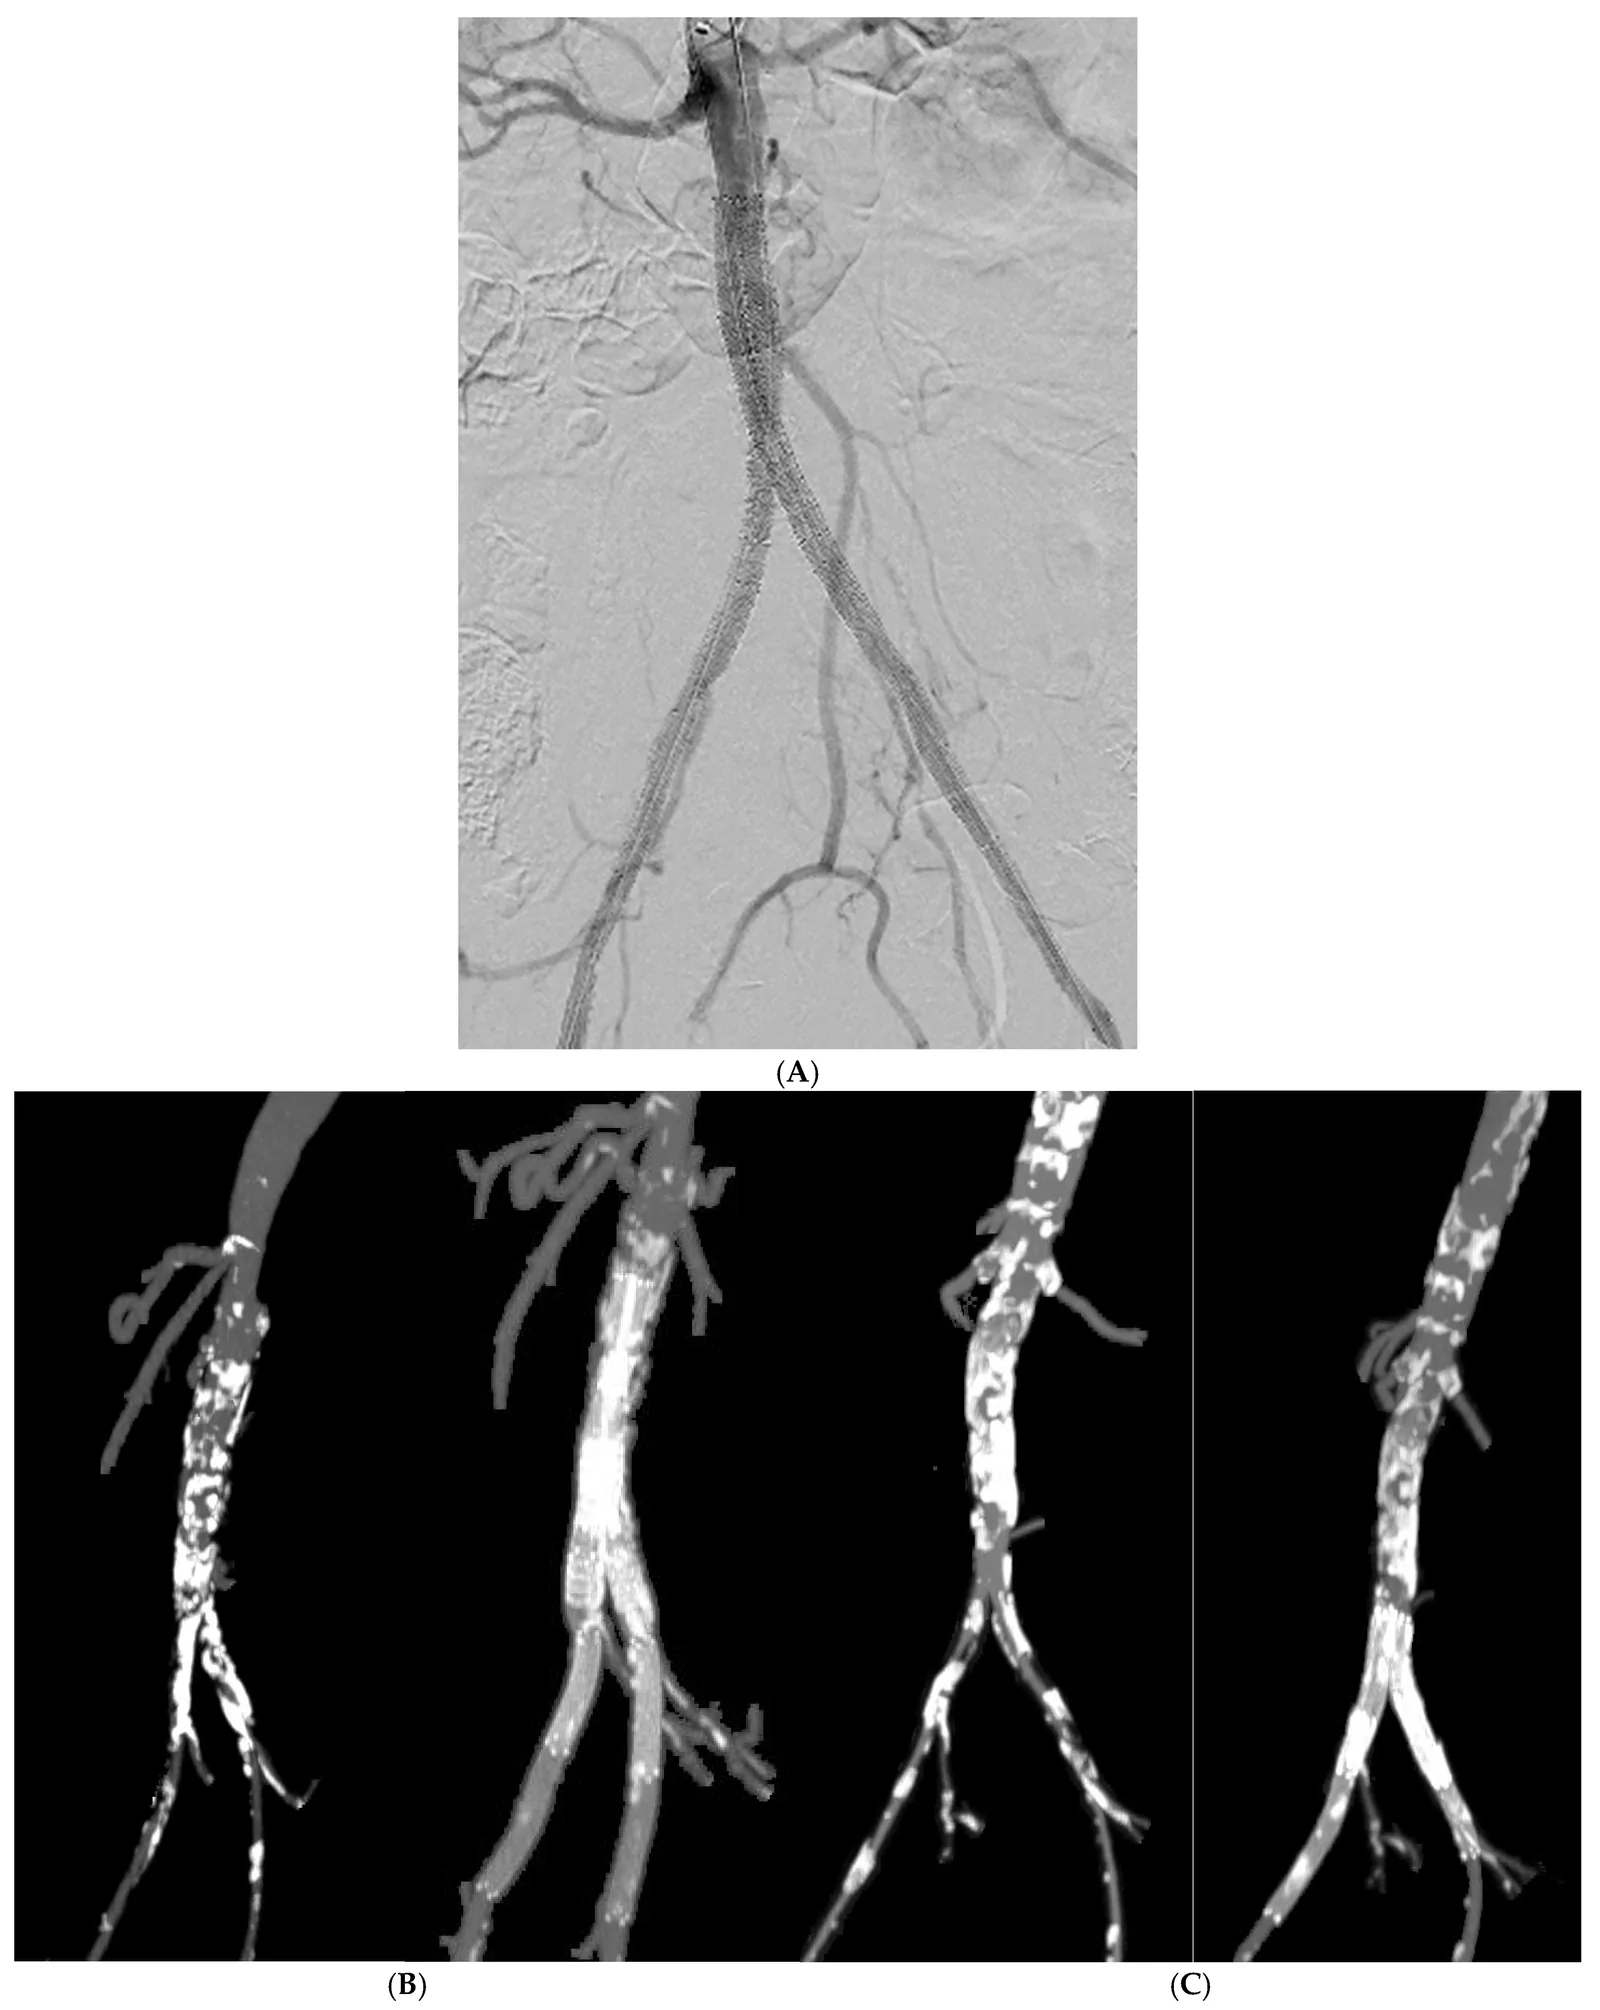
(A) The kissing stent technique consists in deploying two stents simultaneously in a kissing fashion, landing proximally in the distal aorta and distally in the common iliac arteries. Representative preoperative and postoperative CT angiographic reconstructions of the aorto-iliac bifurcation when using covered stents (B) and bare-metal stents (C), respectively.
